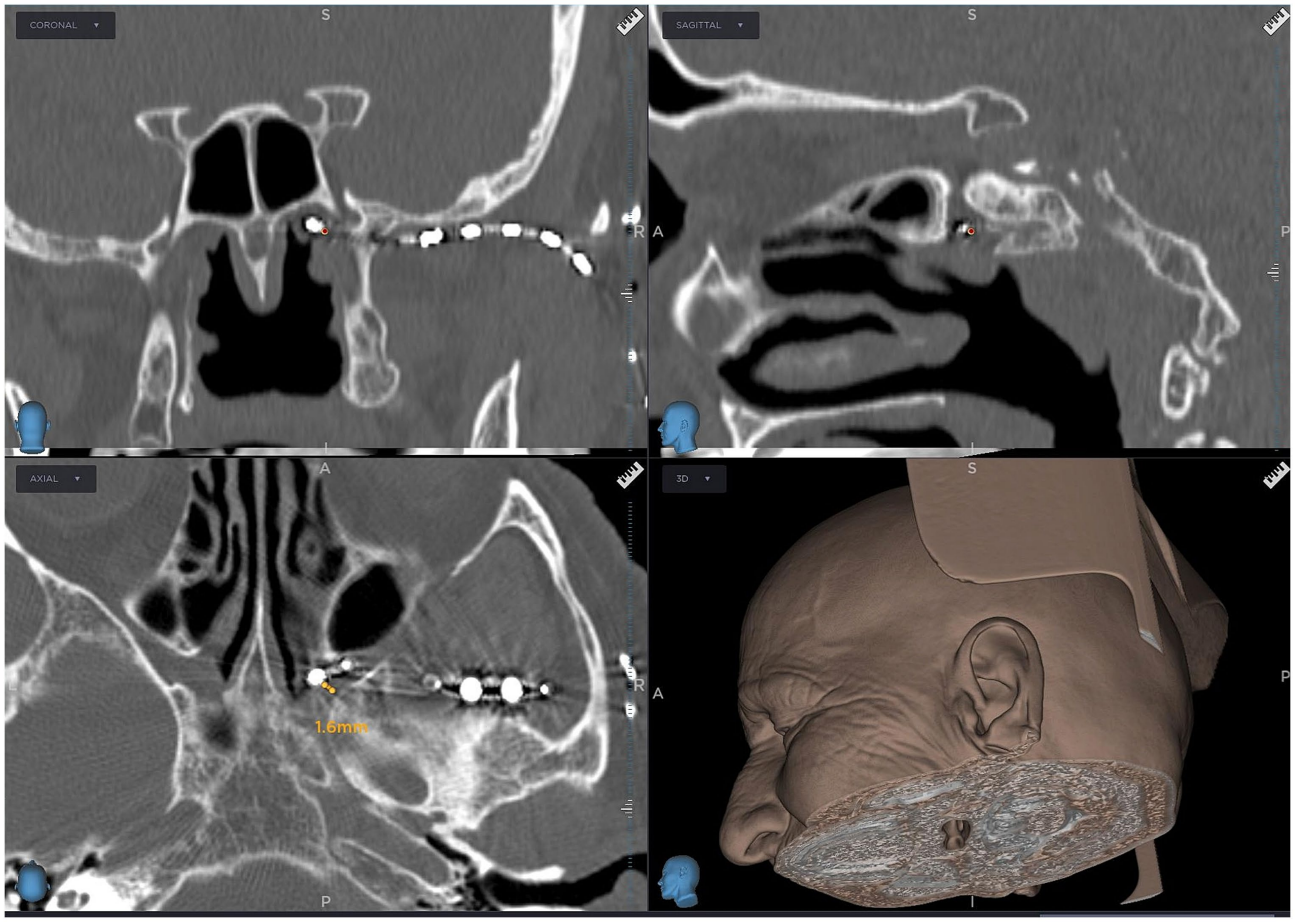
Computed tomography scan showing an 8-contact electrode lead implanted in the pterygopalatine fossa near the sphenopalatine ganglion, guided by neuronavigation (Stealth, Medtronic, United States).
